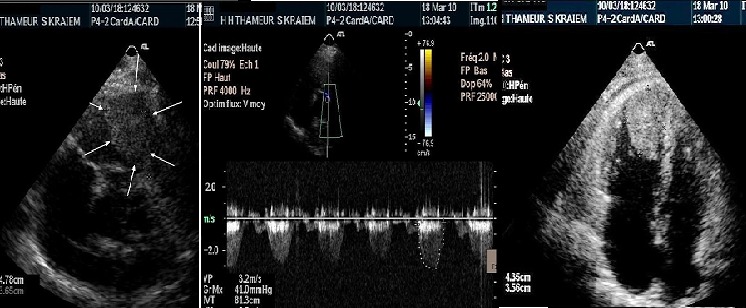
Echocardiography showing the right ventricular mesuring 43 cm long axis with important pulmonary artery obstruction assessed by the pulmonary mean gradient
